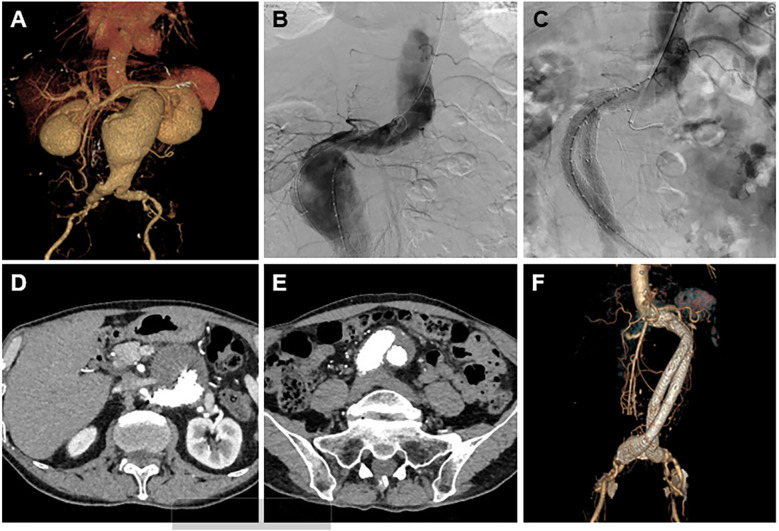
A result of the treatment and follow-up using the Kabedon technique for endovascular management of severely angulated neck infrarenal abdominal aortic aneurysms. (A) Preoperative CTA 3-dimensional volume-rendering reconstruction showing a proximally angulated neck, infrarenal aneurysmal neck, and abdominal aortic aneurysm sac. (B) Intraoperative angiography showing the stiff guidewire status, herein called “Kabedon.” Tension was maintained through the guidewire along the large curvature of the AAA. (C) Completion angiography demonstrates abdominal aorta and limb reconstruction without endoleaks. (D,E) The latest follow-up CTA showed no endoleaks, and migration occurred at the proximal and distal stent grafts. (F) A CTA scan 1 year after endovascular repair showing exclusion of the aneurysmal sac with no evidence of type Ia or III endoleak or graft limb occlusion.
